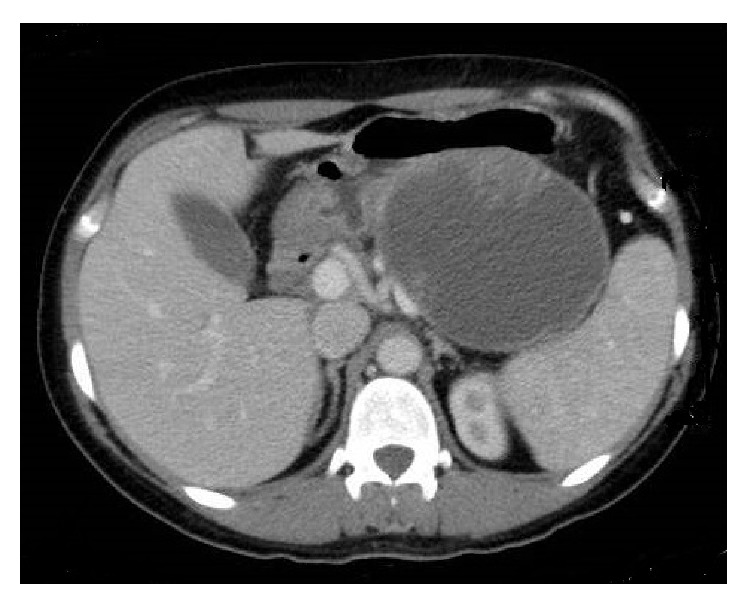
Computed tomography of the abdomen showing a large cystic mass with solid components in the body-tail of the pancreas (case number 13).
